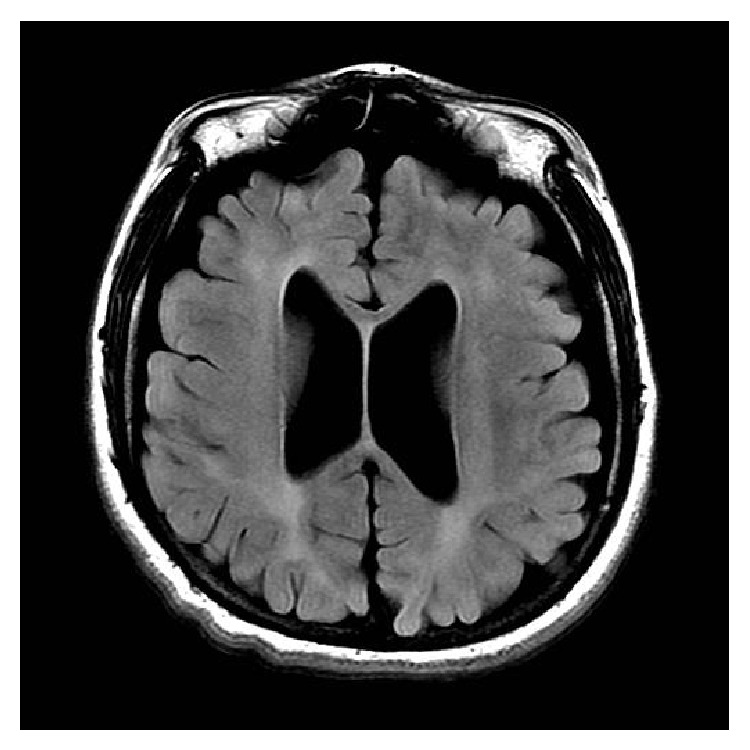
Axial MRI view of the brain at the age of 17 showing bilateral diffuse symmetric increased FLAIR signal in the subcortical and periventricular white matter with diffuse atrophic changes involving the supratentorial brain. Axial FLAIR image performed on a 3T MR GE scanner with TR: 9002, TE: 124.7.
